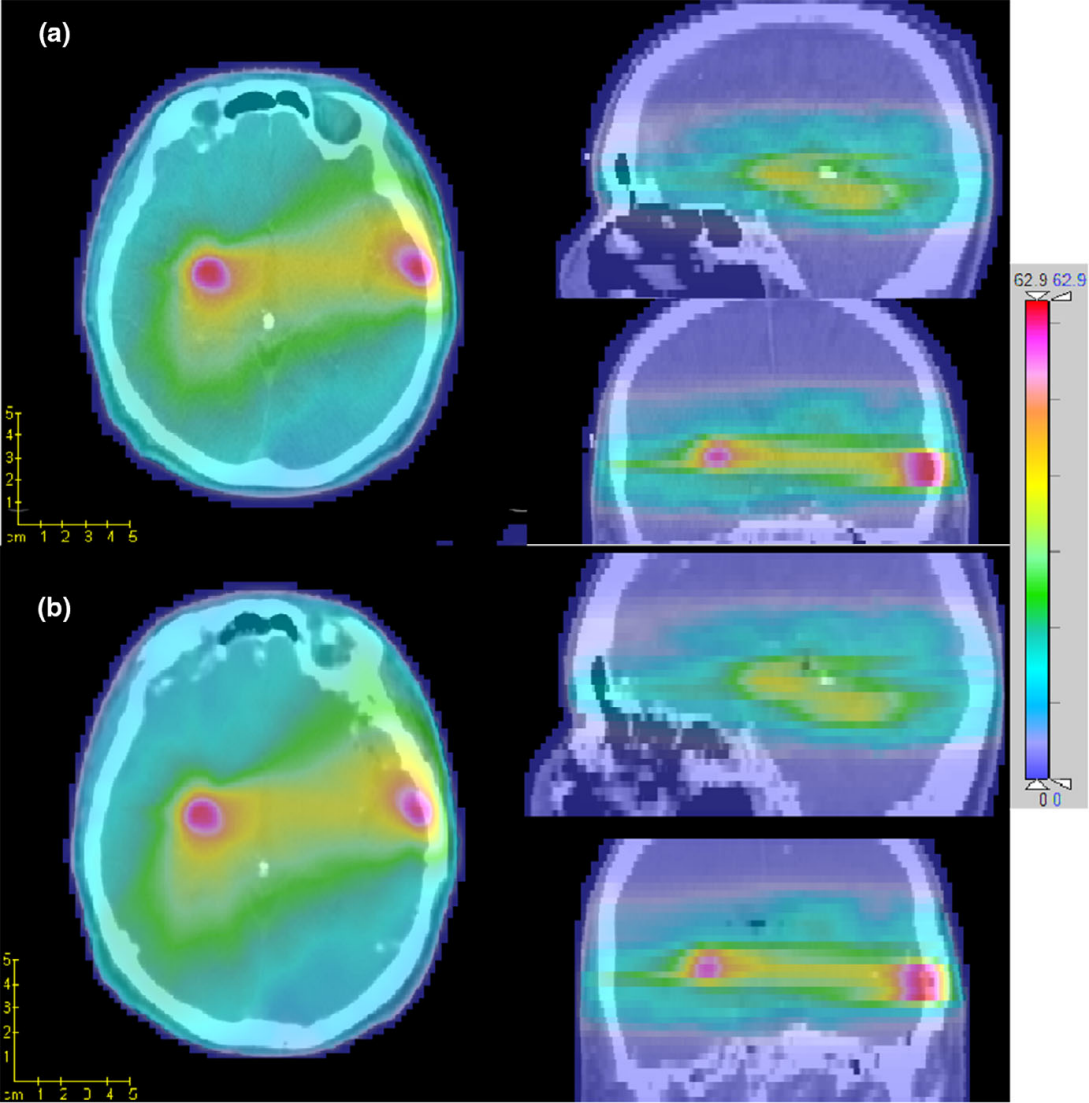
Dose distribution of a clinical VMAT plan calculated on planning (a) and synthetic (b) computed tomography.
